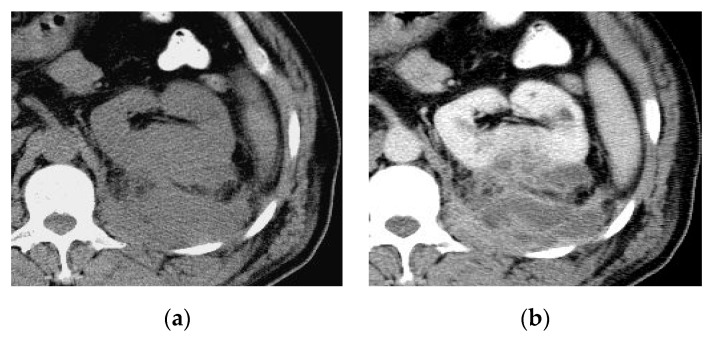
Left renal and perirenal abscess. Axial (a) non-contrast and (b) post-contrast CT images show multilocular cystic lesion at the posterior aspect of the mid-zone of the left kidney with enhancing septa, it extends to the posterior perirenal space involving the left crus of the diaphragm and posterior abdominal wall.
